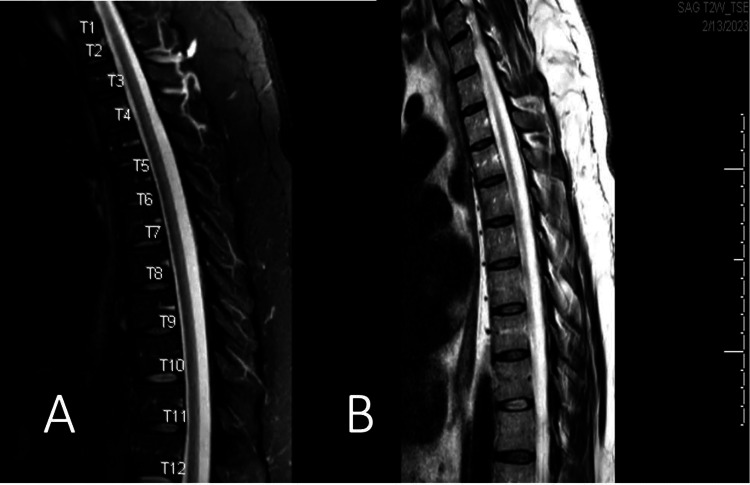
MRI of the thoracic spine without and with contrast revealed T2 hyperintensity of the dorsal columns from T3 to T11, most prominent at T7, without enhancing lesions. A. T1-weighted image B. T2-weighted image
